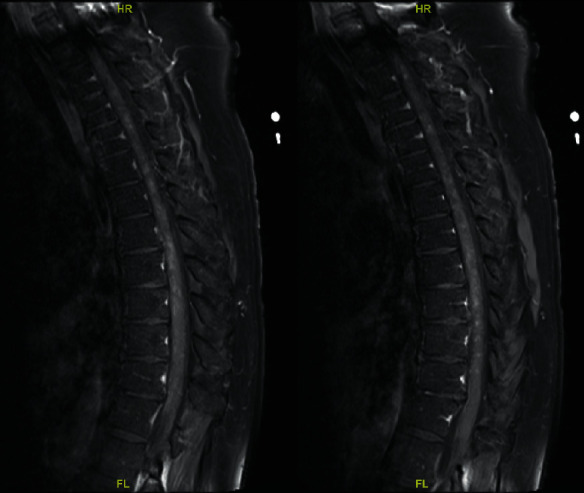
MRI spine T1-weighted sagittal images showing contrast enhancement.
